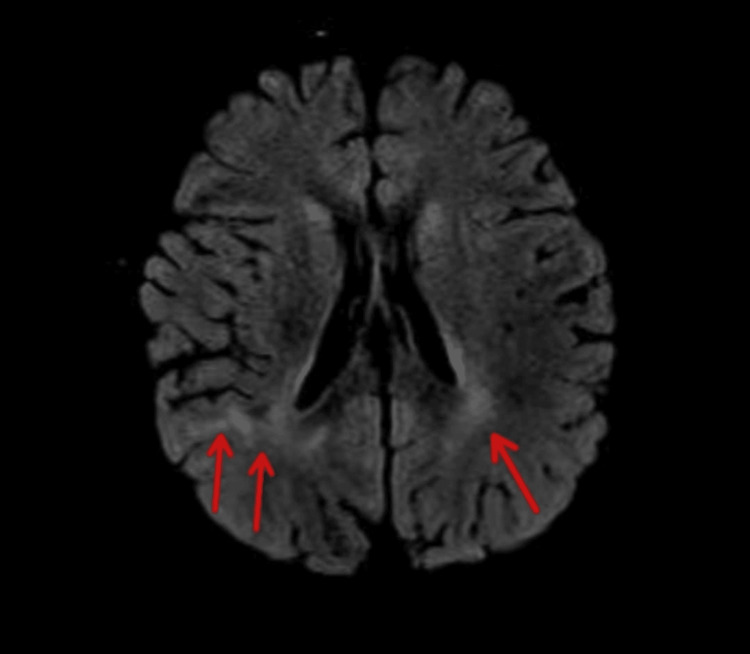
Brain MRI axial view with FLAIR sequence showing multiple posterior hypersignal intensities involving the panventricular, cortical and subcortical areas. FLAIR: fluid-attenuated inversion recovery
